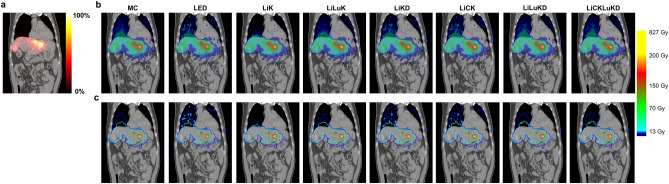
Coronal view of (a) the SPECT/CT fusion image, (b) the absorbed dose map and (c) isodose contour of a sample patient for MC and different VSV methods.
